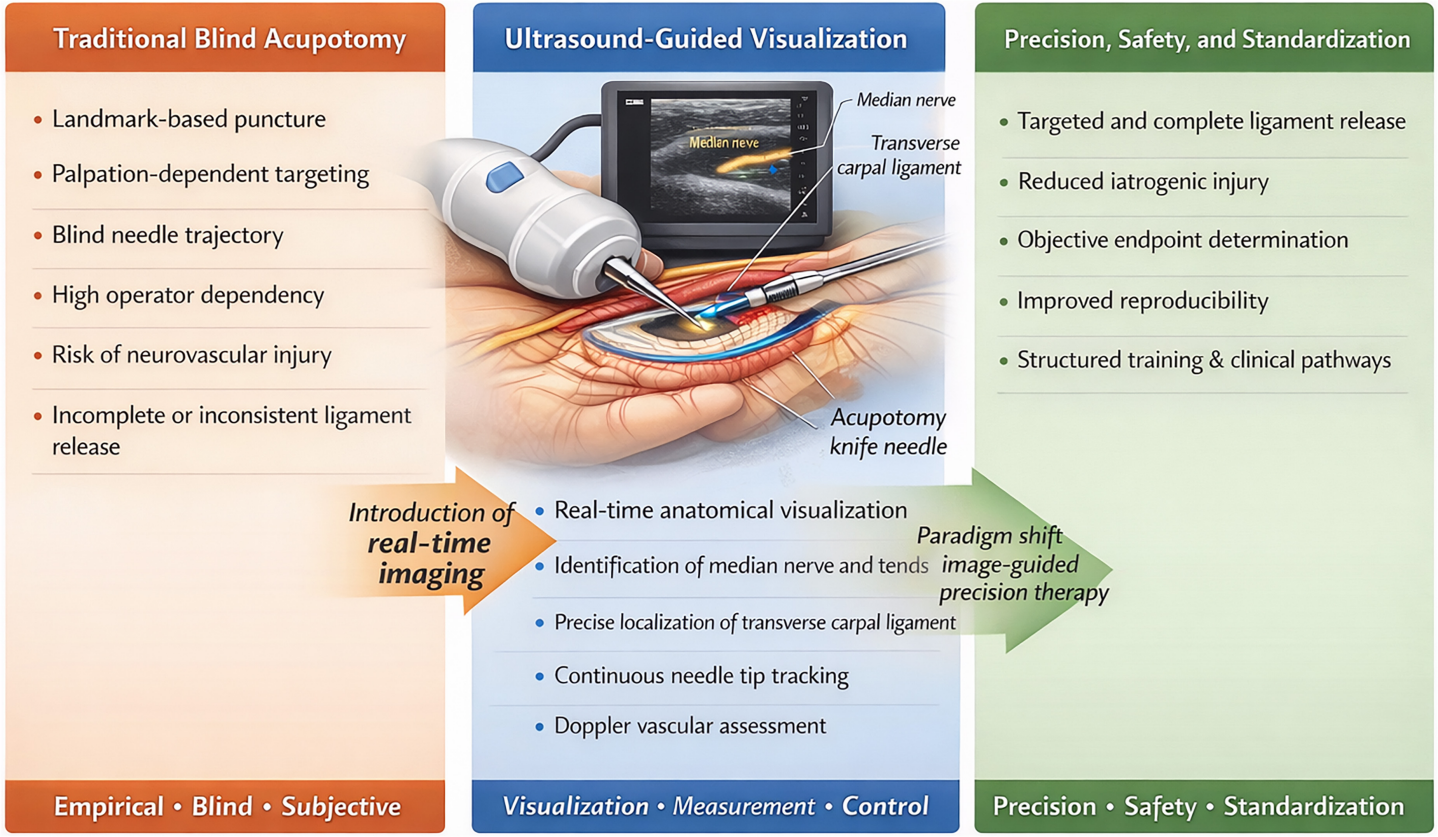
Paradigm shift from blind acupotomy to ultrasound-guided precisious intervention in carpal tunnel syndrome.
